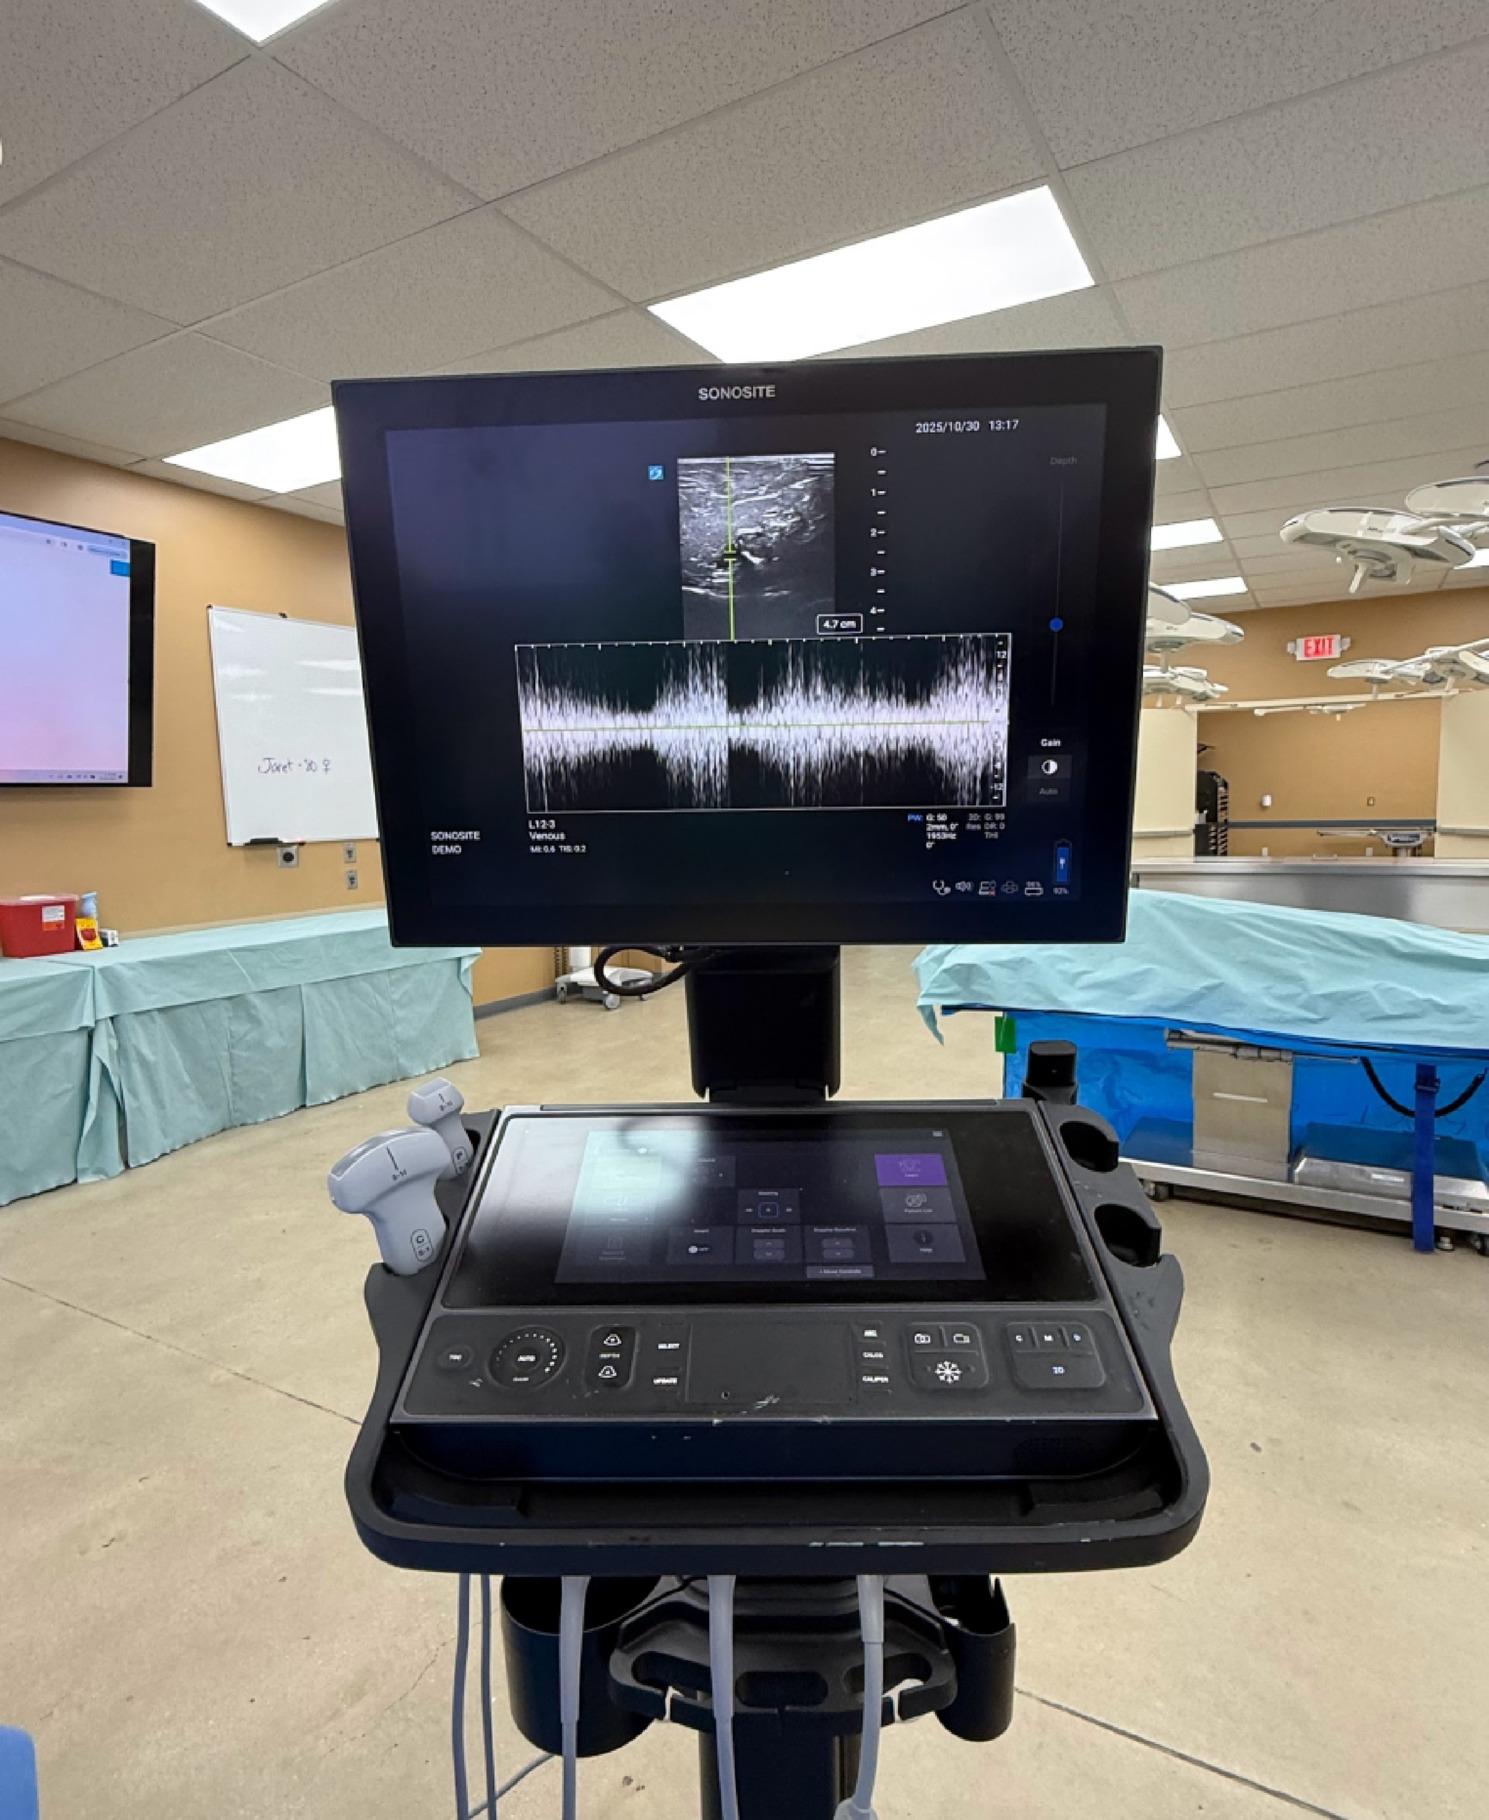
Doppler flow during a fluid bolus through a correctly placed IO
